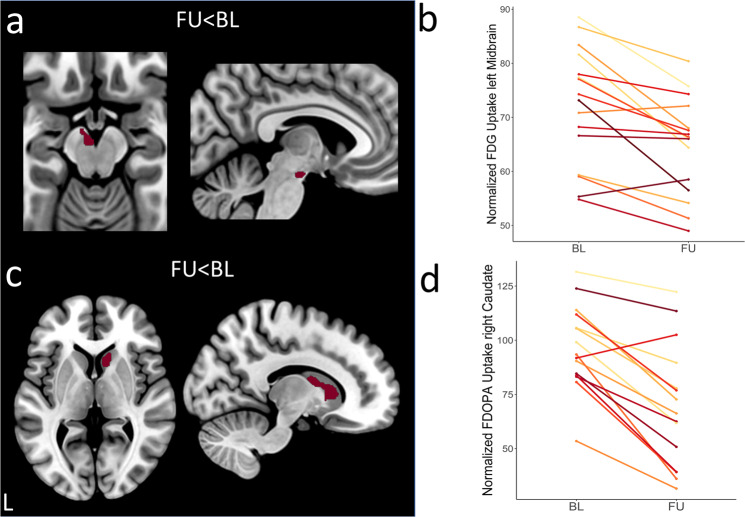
Direct comparison of FDG and FDOPA uptake between follow-up and baseline. Patients showed significantly reduced FDG uptake in the left midbrain (a) and significantly reduced FDOPA uptake in the right caudate (c) at follow-up visits compared to baseline. Both clusters are displayed in binarized form. Results were obtained by applying the above stated contrasts, thresholded at *p*_FWE _< 0.05 cluster-level corrected with SVC for midbrain (a) or striatum (c), respectively. Progression plots show the decrement of tracer uptakes between both visits for each patient individually for left midbrain FDG uptake (b) and right caudate FDOPA uptake (d). Progression plots contain extracted normalized FDG and FDOPA values with proportional scaling. BL = baseline, FU = follow up, HC = healthy controls.
